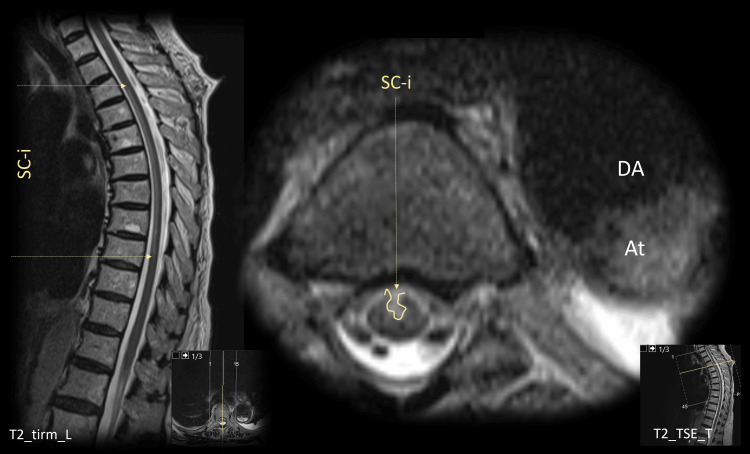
Spinal cord MRI At - Aortic thrombosis; DA - Descending Aorta; SC-i - Spinal Cord Infarction
